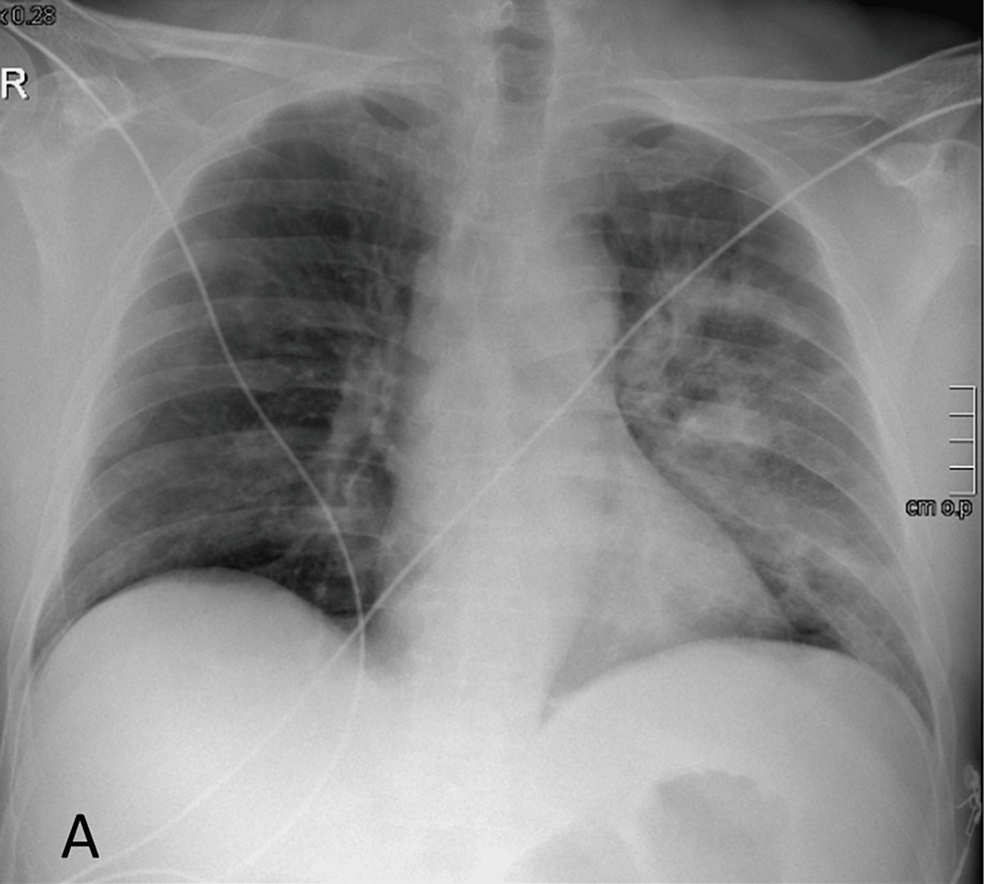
X-ray of the patient in case 3 X-ray at presentation showing left alveolar infiltrates.
